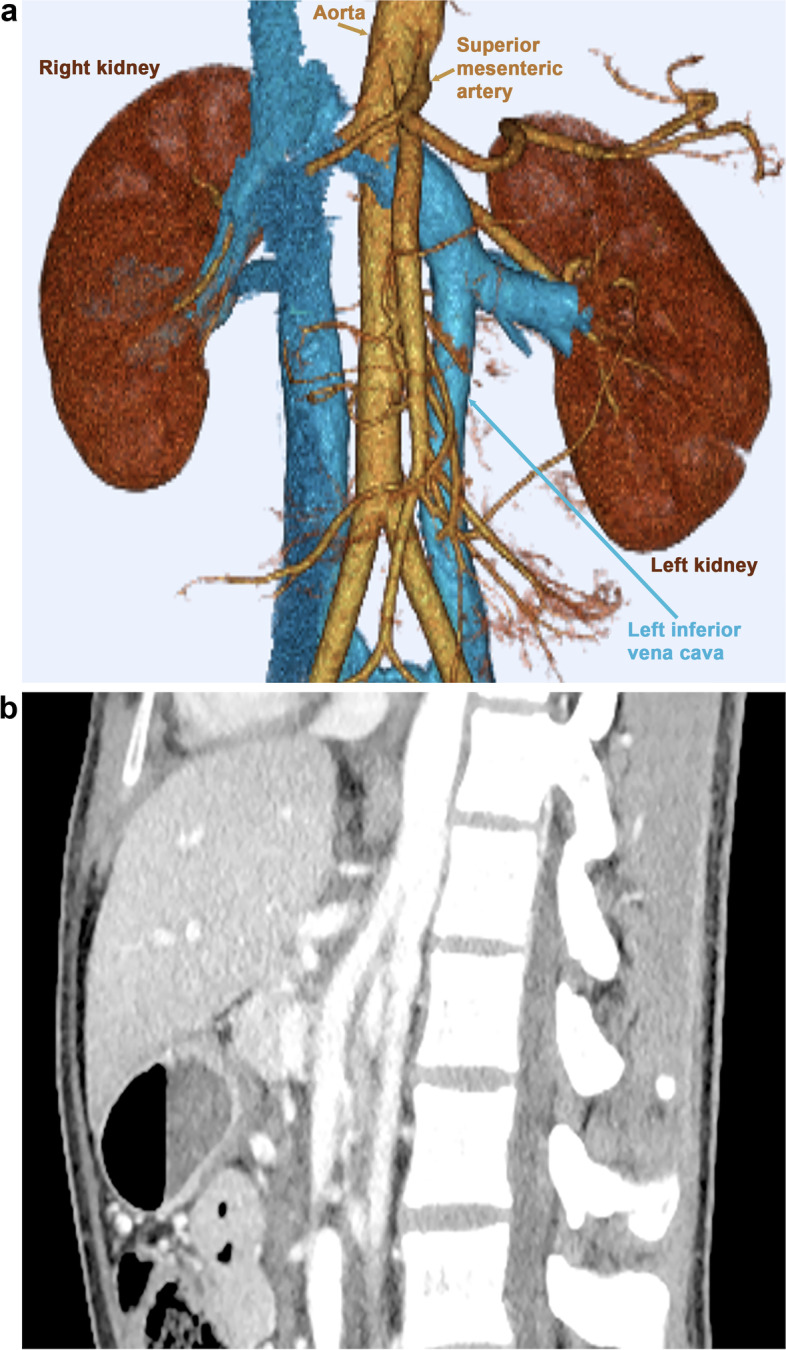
(a) A three-dimensional contrast-enhanced computed tomography image. Double inferior vena cava and narrowing (arrowhead) of the left renal vein were observed after interflow of the left inferior vena cava. (b) The aortomesenteric angle on sagittal reconstruction of the computed tomography image was 14.7°
